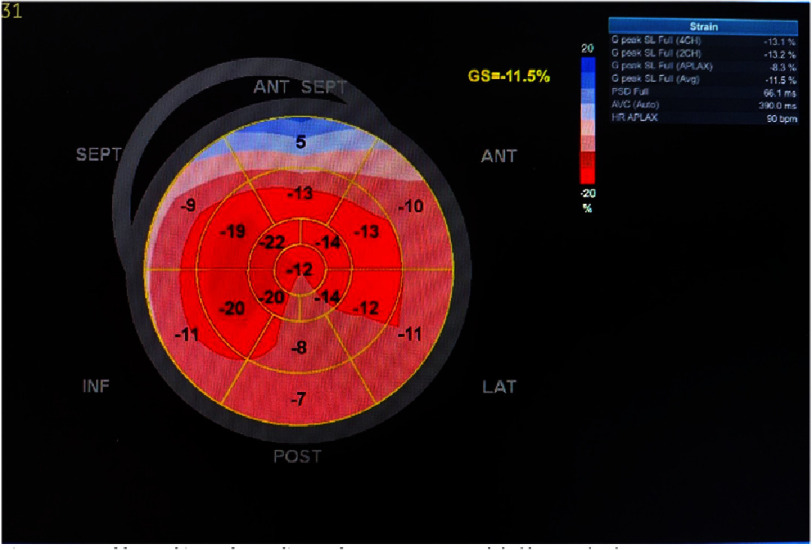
Speckle-tracking echocardiography (STE). Average global longitudinal strain (GLS) was -11.5%.
